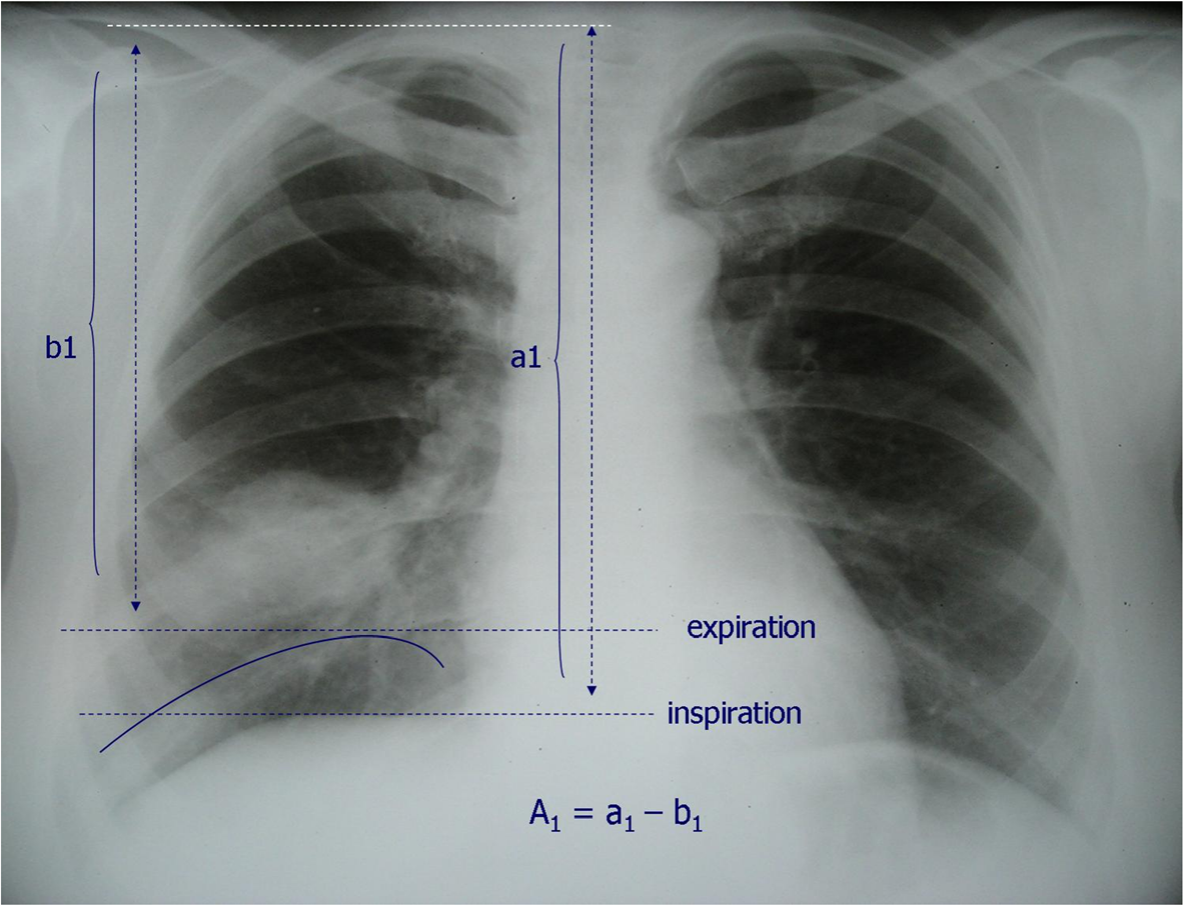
Radiographic measurement of diaphragm movements.
